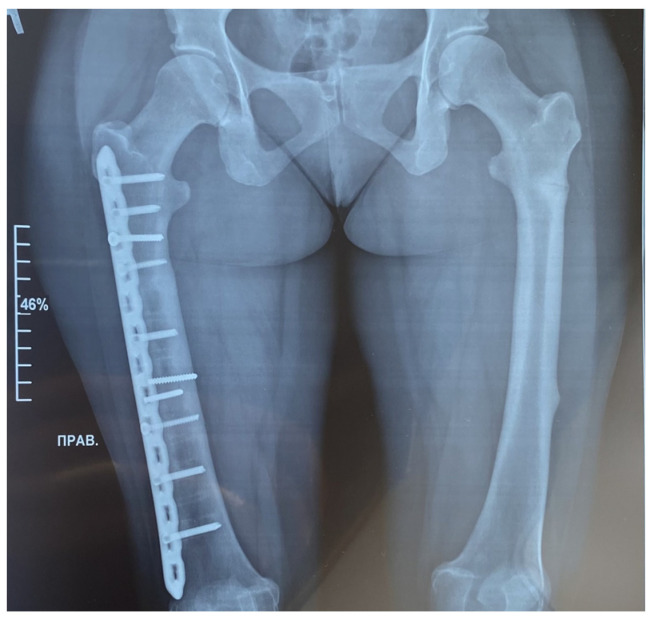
Radiographic examination of the femur bones was conducted using both frontal projections. On the right side, a fracture of the femur was treated with the insertion of a metal osteosynthesis structure (MOS). The position of the metal structure is satisfactory, without displacement. The fracture line is not clearly discernible, yet sufficient consolidation has occurred. Transverse shadows of the previously installed MOS are visible. The axis of the limb remains unchanged. A transverse fracture without displacement is evident on the left side of the diaphysis on the posterior–external surface. The fracture line is clearly visible. Consolidation is poorly expressed. The femur bones show a pronounced thickening of the endosteum, reaching a maximum of 9 mm (bone canal narrowed).
